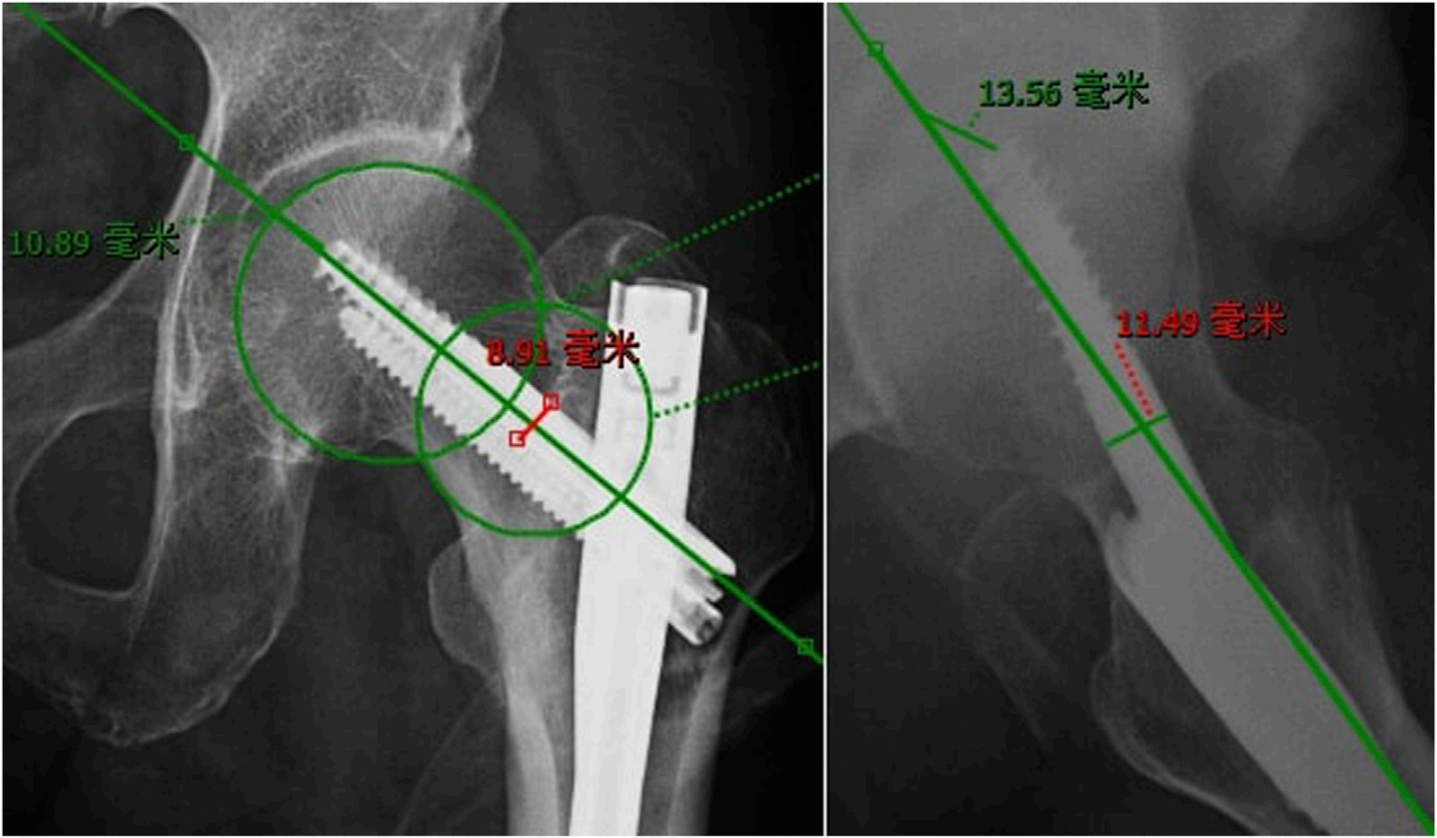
Measurement of the tip apex distance on the anteroposterior and lateral radiographs (mm).
